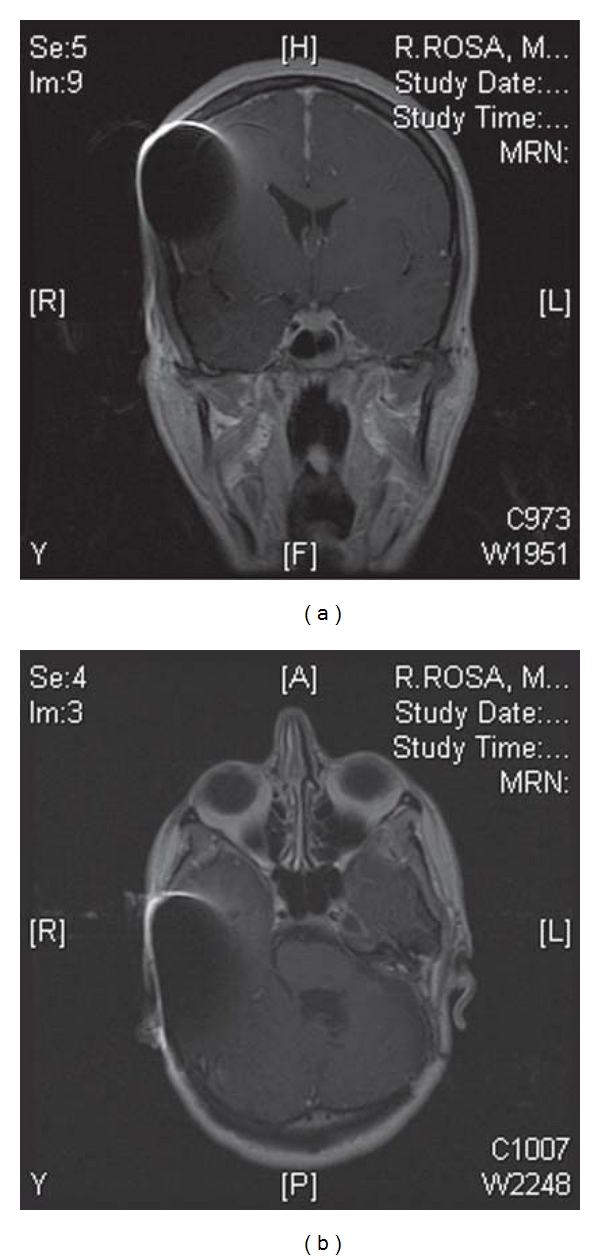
MRI followup at 1.5 T of a patient with surgically removed VS and cochlear implantation (Pulsar CI 100, Med-el).
